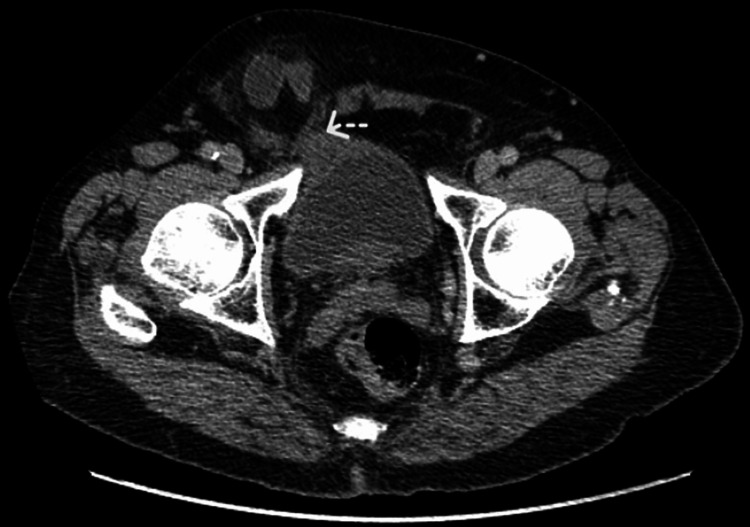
A CT scan image showing a urinary bladder adherent to the hernia sac (→)
